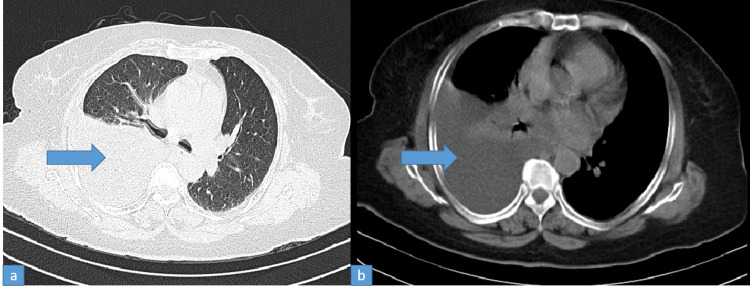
CECT thorax showing right pleural effusion - lung (a) and bone (b) windows CECT: contrast-enhanced computed tomography
